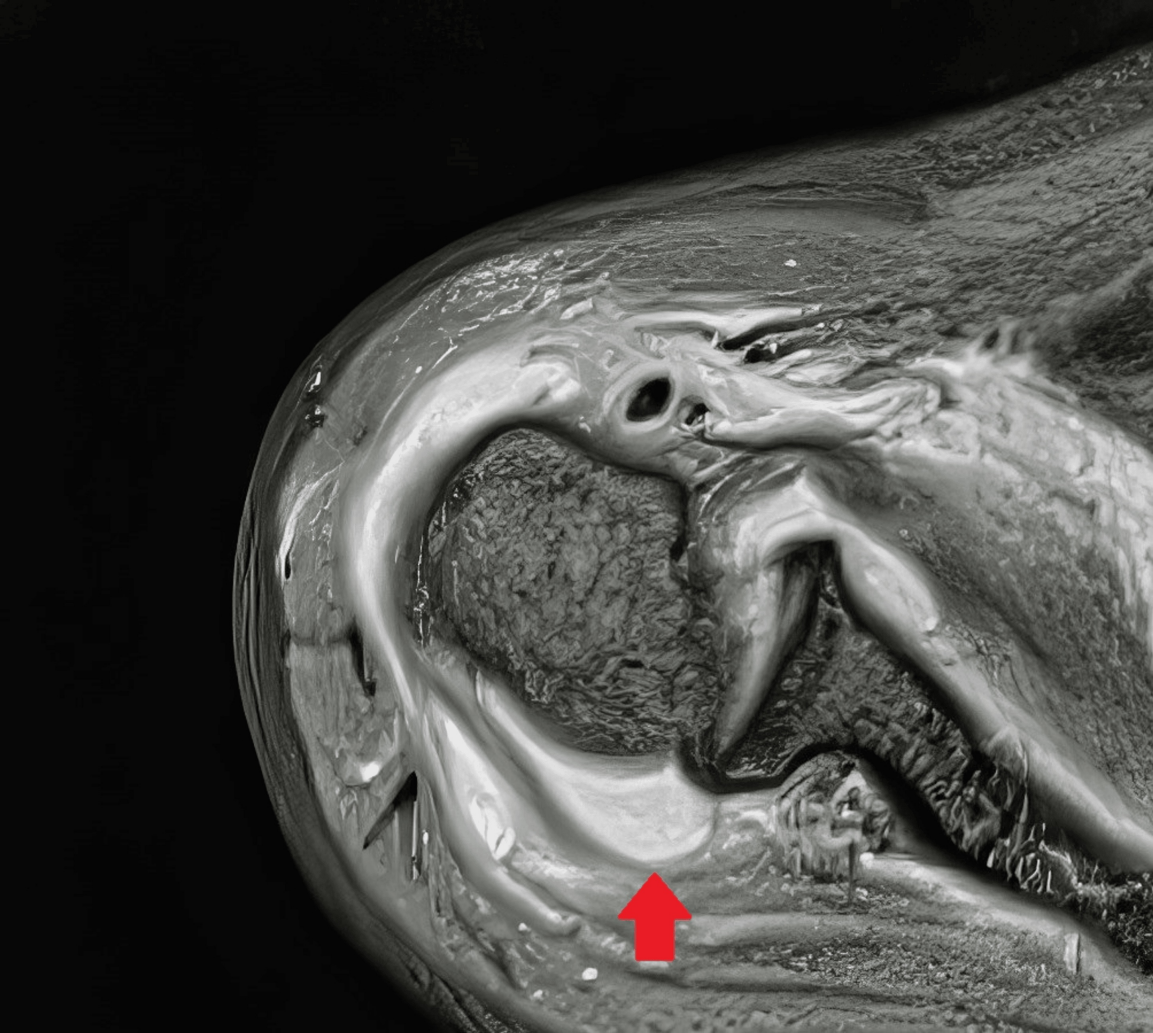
MRI of the right shoulder showing collection in the joint (red arrow) MRI: magnetic resonance imaging
